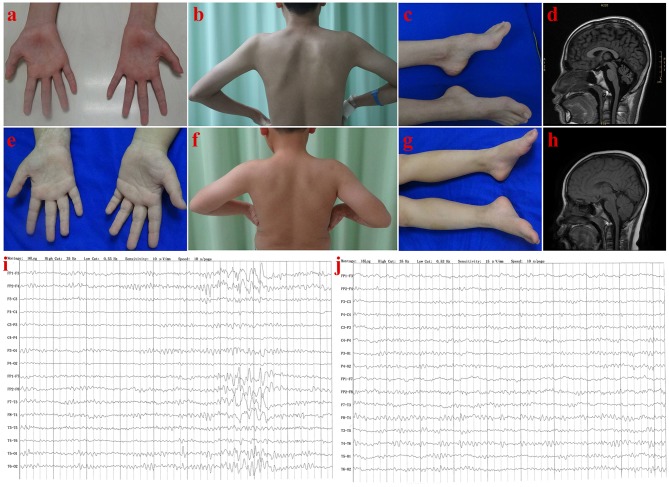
Clinical features of patients in Family 1. Patient III-1: Muscle atrophy in the thenar muscle, interosseous muscle of hands (A), and shoulder girdle muscle (B); bilateral pes cavus (C); diffuse cerebellar atrophy on T1-weighted MRI (D); EEG showed increased slow waves, paroxysmal sharp-slow wave in the frontal and temporal region (I). Patient III-2: No muscle atrophy was found in hand (E), and shoulder girdle muscle (F), with normal appearance of feet (G); T1-weighted MRI of the brain (H), and EEG (J) were normal.
